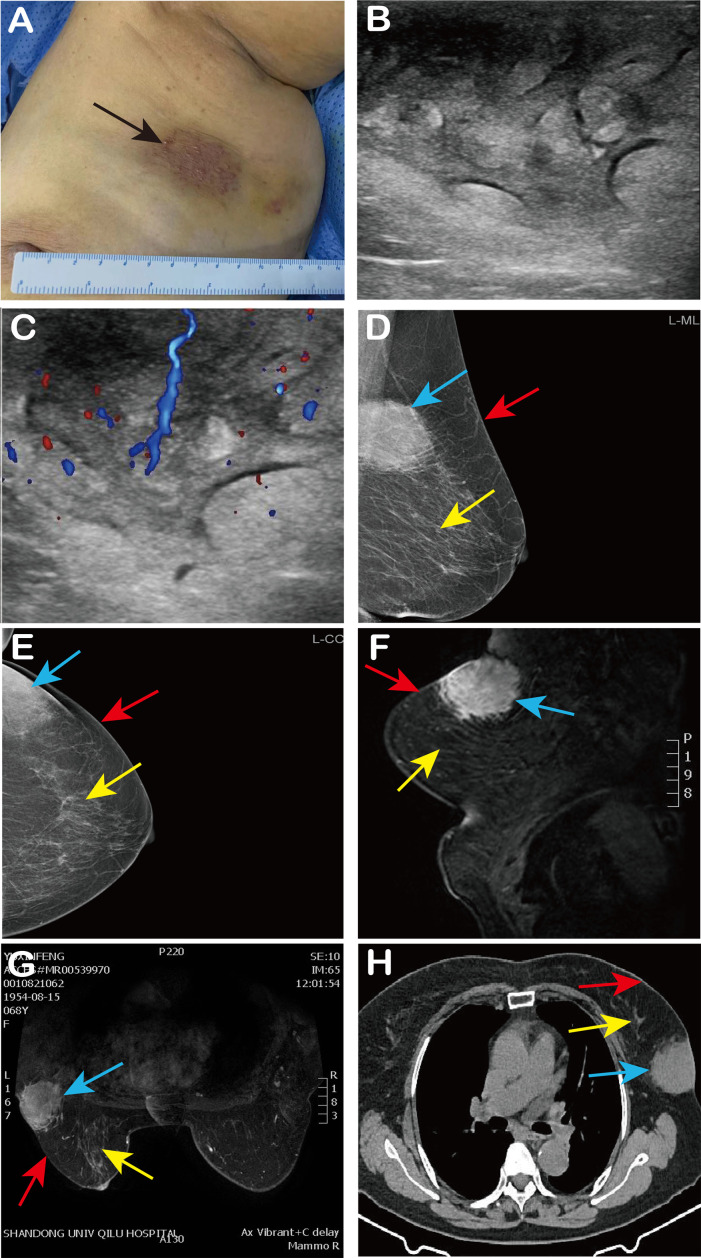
Patient’s clinical findings and imaging findings. (A) We observed red and purple skin changes on the surface of the mass (black arrow); (B, C) Ultrasound showed an echo-enhanced mass of 8 cm × 5 cm × 3 cm in the left breast, with cobblestone changes and abundant internal blood flow signals; (D, E) Left breast mammography (MLO, CC) revealed an asymmetric dense shadow in the upper outer quadrant of the left breast; (F, G) MRI of the breast indicated an abnormal signal in the glandular tissue at the posterior edge of the upper outer quadrant involving the skin; (H) CT shows a soft tissue density mass in the left breast with spiculated margins and connection to the skin. In Figures (D–H), the blue arrow indicates the lump, the red arrow indicates the breast skin, and the yellow arrow indicates the breast tissue.
